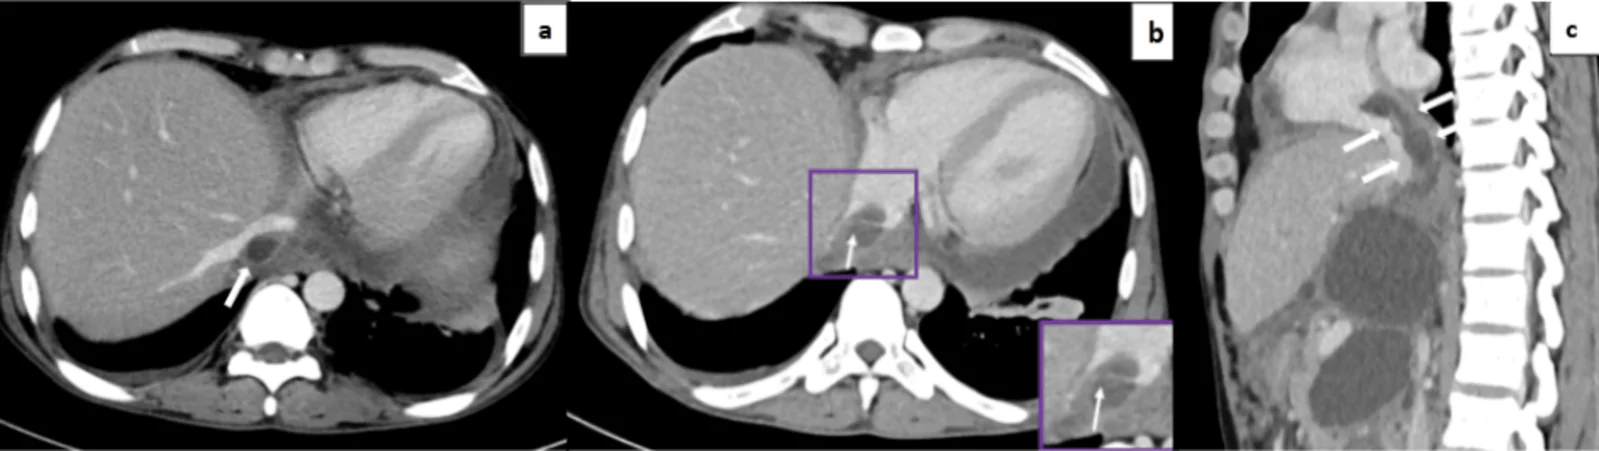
Contrast-enhanced CT of the thorax and abdomen (a) Axial section showing the collection (white arrow) adjacent to the inferior vena cava; (b) Axial section at the caval-atrial junction showing the focal full-thickness caval wall defect (white arrow) through which the collection herniates into the lumen, with its fibrous capsule bridging the defect and separating the contents from the opacified blood pool; (c) Sagittal reformat showing longitudinal extension of the collection along the periadventitial plane of the inferior vena cava and its intracavitary component within the right atrium (white arrows)
